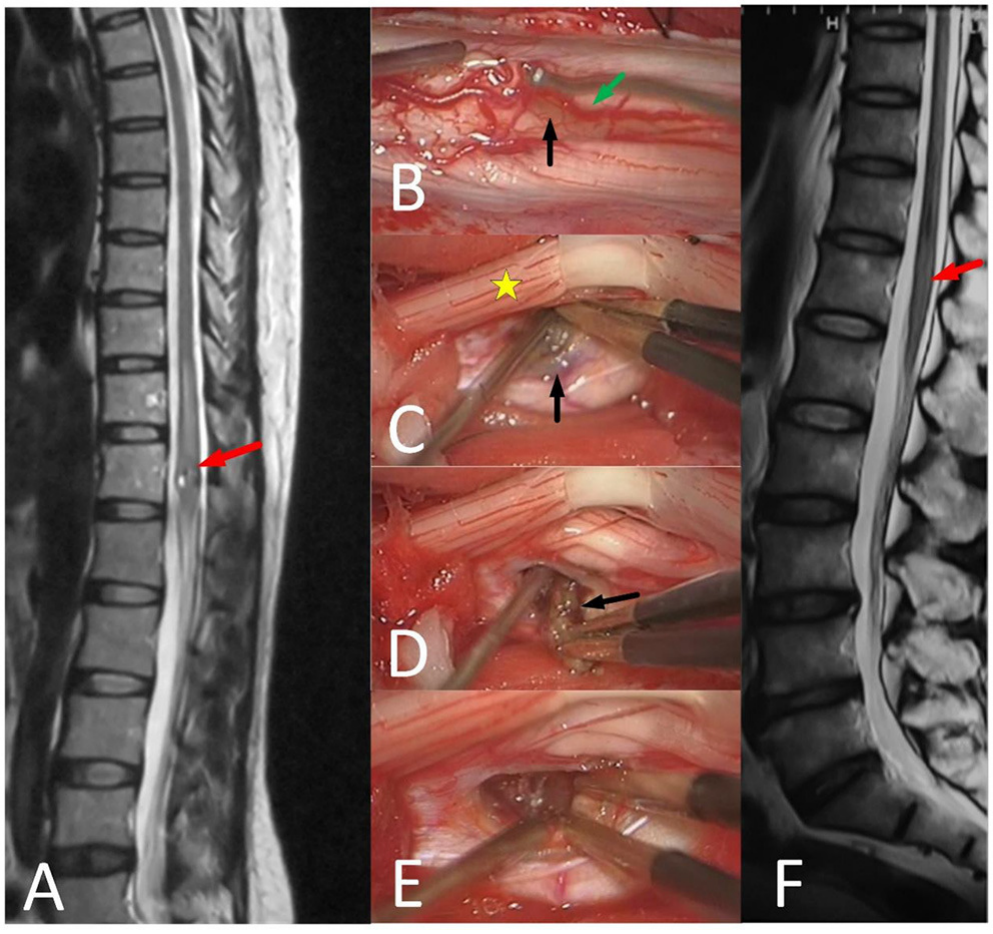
51-year-old female with unsteady walking for 6 months and urinating difficulty for 3 days (mALs = 11 points, severe disorder, type B1), was treated by ERS. (A) Preoperative spinal MRI examination revealed ISCH in ventral conus (red arrow). (B–E) A The brown lesion on the spinal cord surface (black arrow) could be seen after pushing posterior nerve roots (yellow star) aside and the artery (green arrow) was protected carefully. (F) MRI reexamination showed no residual or recurrence at 3 years after surgery and the mAS was 10 points at the last follow-up.
